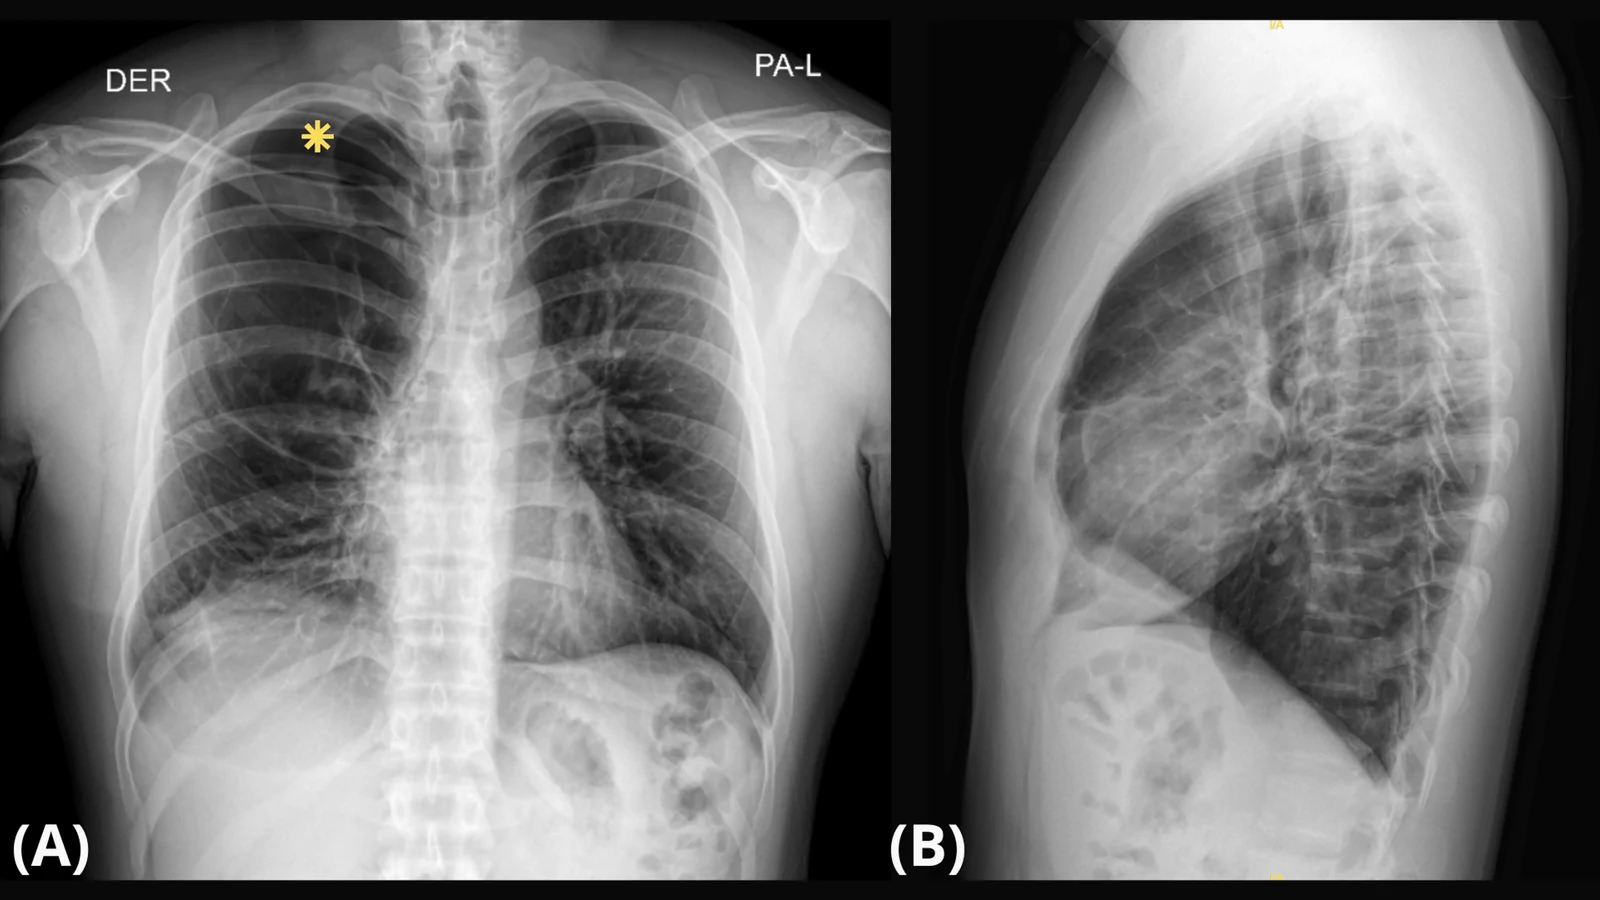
Follow-up chest radiographs seven months after surgery. (A) Posteroanterior and (B) lateral chest radiographs obtained seven months after the right upper lobectomy show no consolidation, normal pulmonary vascularity, and free costophrenic angles. A small residual apical space is seen on the right (*), an expected finding after upper lobectomy, in an asymptomatic patient.
